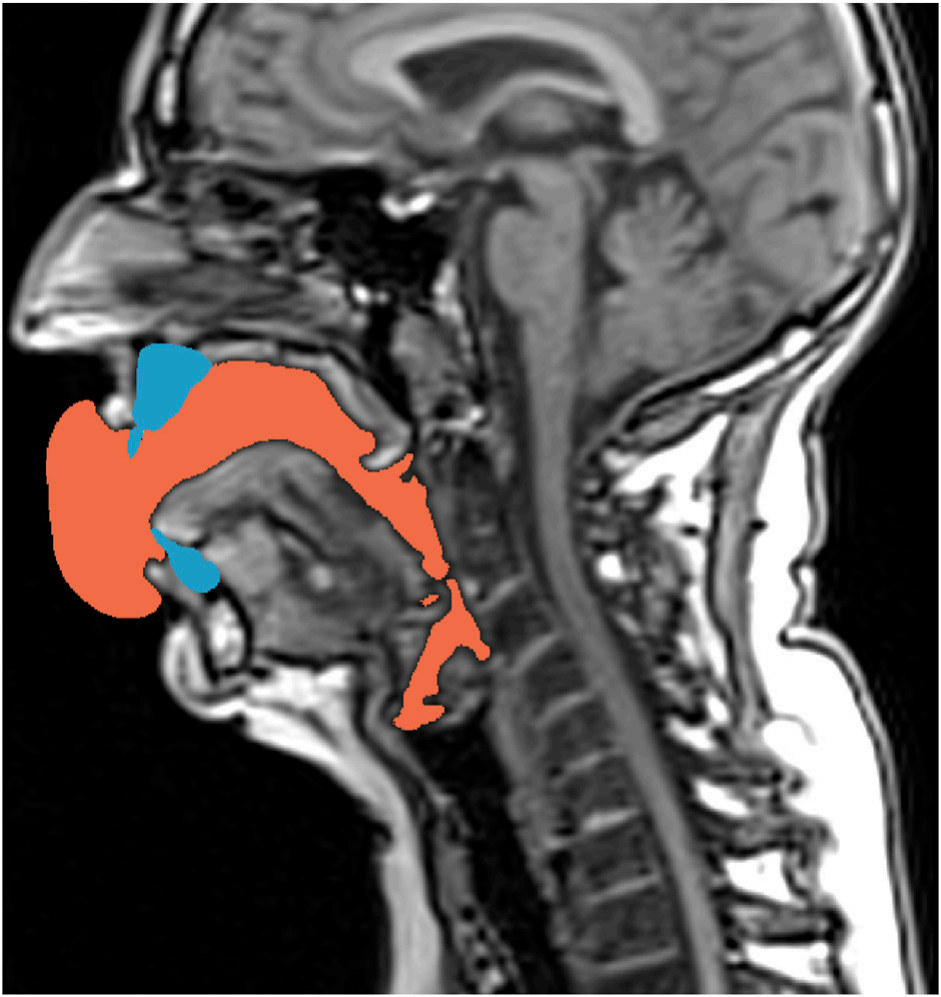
Exemplarily shown for Belting /aː/ (A♭3), the mid-sagittal slice of the MRI stack shows the air-filled cavities in black color. Additionally, the post-processed teeth models (blue) and the finally derived segmentation of the vocal tract cavity used in the FE calculation (orange)—considering the teeth not shown in the primary MRI data–are overlayed.
